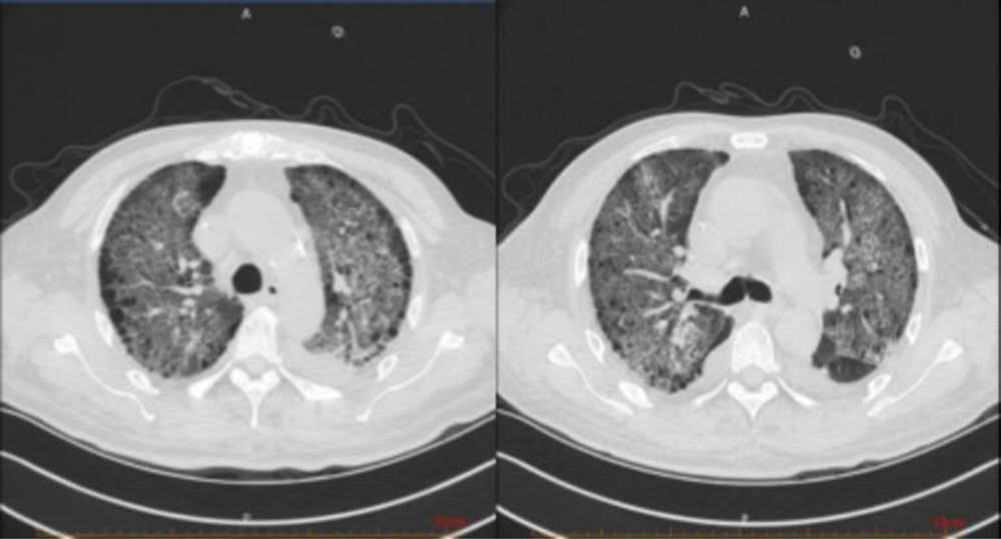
CT of the lung on April 2nd: compared with March 26th, the area of multiple exudative lesions in both lungs has increased compared to before, while the size of some lesions has decreased and the density has decreased. CT = computed tomography.
